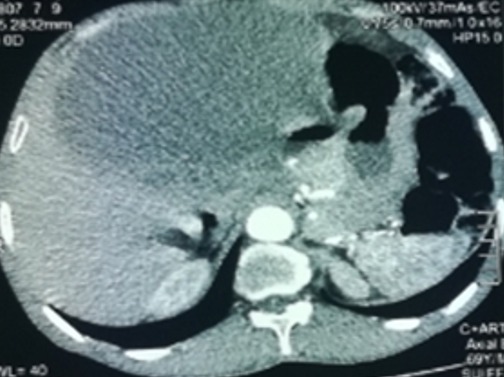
CT image of the hydatid cyst
